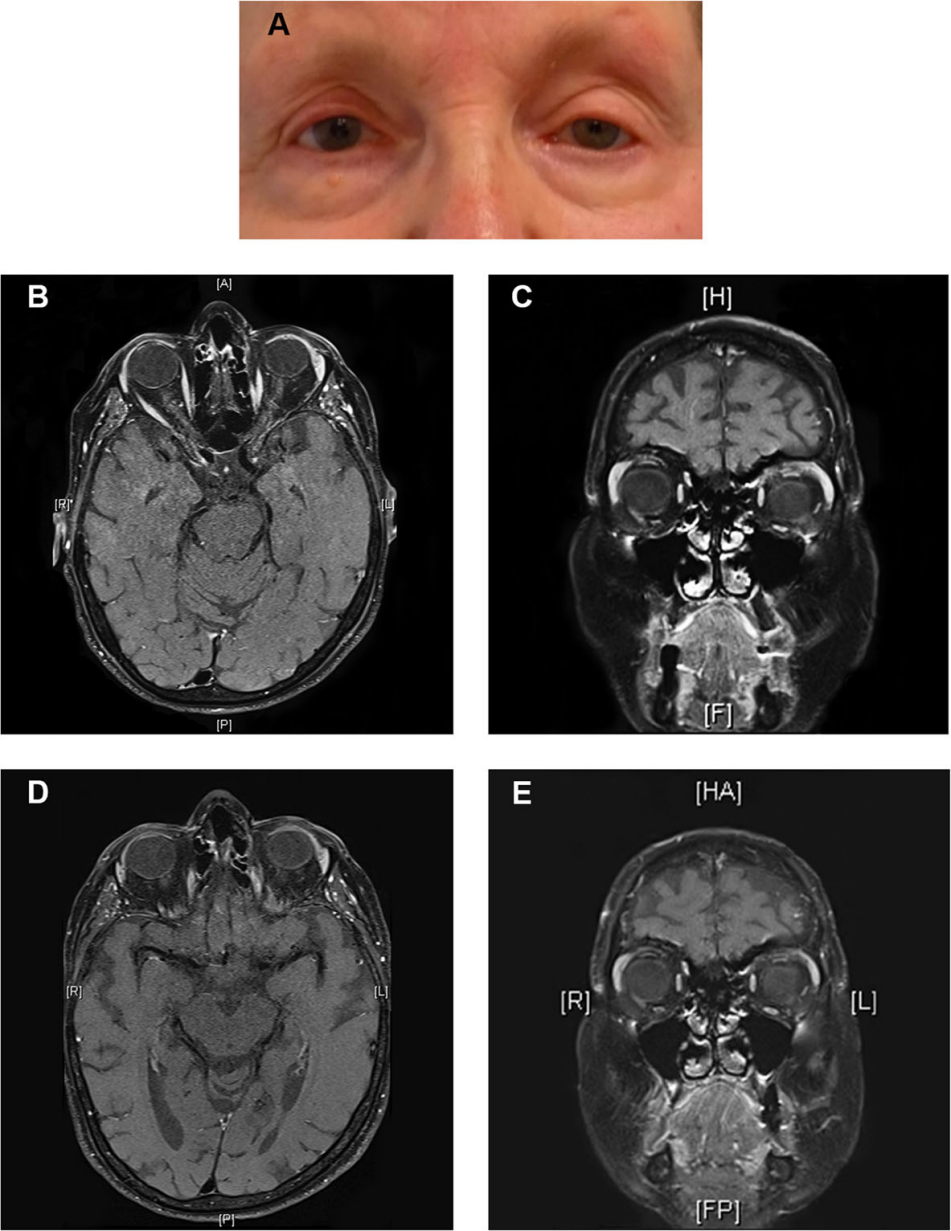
Clinical and radiologic presentation after initiation of antifungal therapy. a shows an en-face photograph of the patient 14 weeks after beginning the antifungal therapy. Axial (b, d) and sagittal (c, e) fat-suppressed T1-weighted and coronal (d) T1-weighted (without fat suppression) MR images showing an almost complete regression of the mass in the left orbit 11 months (b, c) and a complete regression of the mass 24 months (d, e) after the beginning of the therapy
